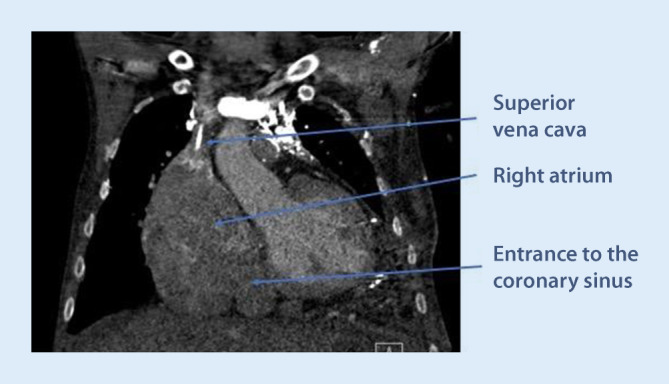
Preoperative cardiac computed tomography showing extreme enlargement of the right atrium, with the coronary sinus (CS) ostium in the usual position in the lower medial corner of the atrium. In such a case, a standard CS catheter often has insufficient support to enter the CS, and larger or stiffer sheaths need to be usedii.The CS ostium may be partly, sometimes completely, covered by the Thebesian valve, which is a thin fibrous structure that may be fenestrated in some cases. Together with the extension of the Eustachian valve, the extension of the Thebesian valve forms a small ridge (Eustachian ridge) running in the right atrium, posterior to and in parallel with the tricuspid annulus. Together with the septal leaflet of the tricuspid valve and the extension of the inferior vena cava valve (the tendon of Todaro), the Eustachian ridge delineates the triangle of Koch, where the AV node is typically located. The ridge is derived from the valve of the embryonic sinus venosus (which is the origin of the valves of the CS and inferior vena cava). The Eustachian ridge may effectively prevent soft cannulation catheters from entering the CS, by deflecting them in a more posterior direction when trying to enter from the atrial side. This problem is best avoided by first entering the right ventricle with the catheter, and then retracting it during counter-clockwise torque. By using this technique, the ridge can actually be of help by guiding the catheter towards the CS ostium once it flips out from the right ventricle through the tricuspid valve.iii.The Thebesian valve can vary in configuration and texture. While it usually does not hamper CS cannulation, it may in some cases be very prominent and impossible to pass using a soft sheath. A prominent Thebesian valve can be seen on cardiac CT or suspected during implant if no or very little contrast enters the CS (Fig. 6). At times, a guidewire can pass through a fenestration, making it easy to advance the wire but impossible to advance the sheath after the wire, since the sheath becomes entangled in the valve around the fenestration. This problem can be overcome by using a steerable solid electrophysiology catheter either on the side or inside the soft sheath. The EP catheter can be manipulated to deflect the valve to allow for the soft sheath to advance into the CS.
